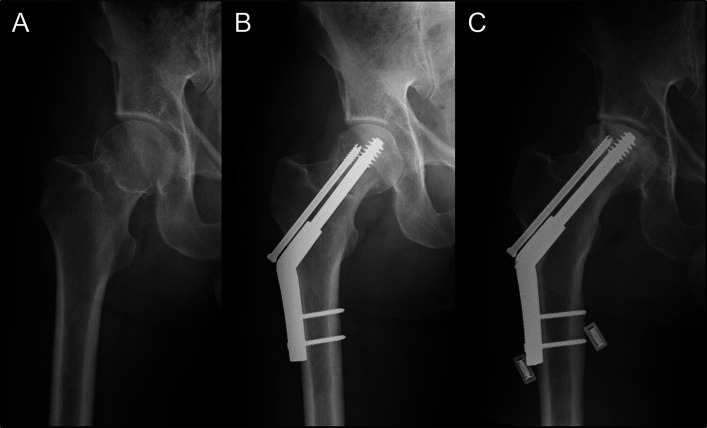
Anteroposterior radiographs of the hip in a 47-year-old man with an intracapsular femoral neck fracture, made immediately following the injury (Fig. 3-A), 6 weeks postoperatively (Fig. 3-B), and 2 years postoperatively, by which time femoral head osteonecrosis had developed (Fig. 3-C).
